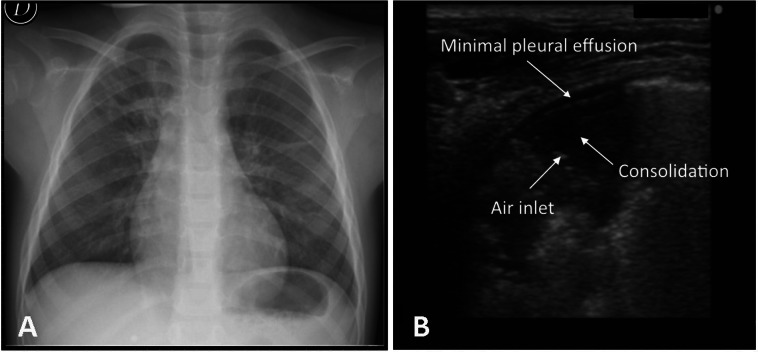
One case of negative chest X-ray and positive lung ultrasound. Negative chest X-ray result for a 4 year old female (A) and evidence of pneumonia in the posterior basal retrocardiac region of left lung by lung ultrasound (B).
